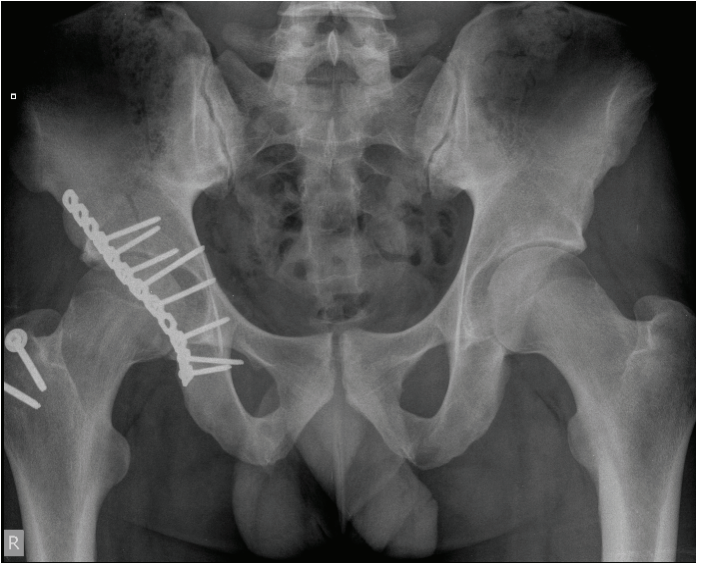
Postoperative X-ray (Case 2).
